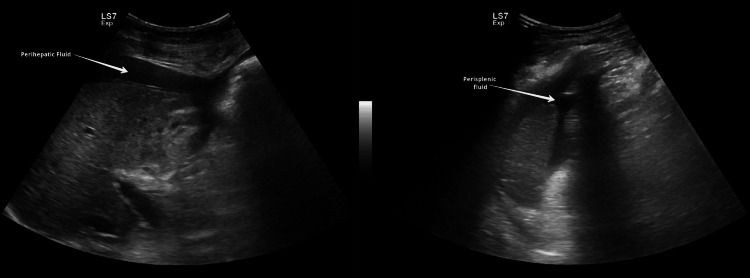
Ultrasound image shows perihepatic and perisplenic fluid suggestive of moderate ascites.
